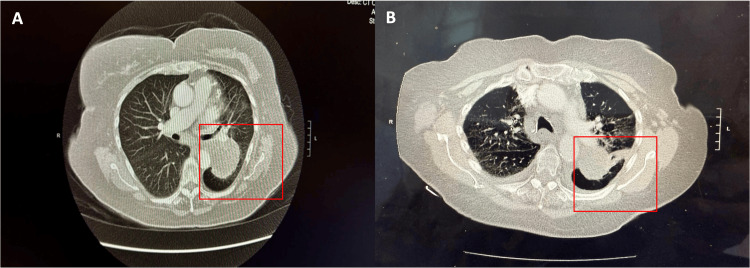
High-resolution contrast-enhanced CT scan of the chest (axial) demonstrating a large mass in the superior segment of the left lower lobe, measuring 6.0 × 5.7 × 5.8 cm (A), without evidence of additional pulmonary nodules, pleural effusion, or significant mediastinal lymphadenopathy. Repeat CT scan chest post two cycles of immunotherapy demonstrates a decrease in size of the mass in the superior segment of the left lower lobe from 5.6 cm to 4.1 cm (B) with some interstitial prominence.
